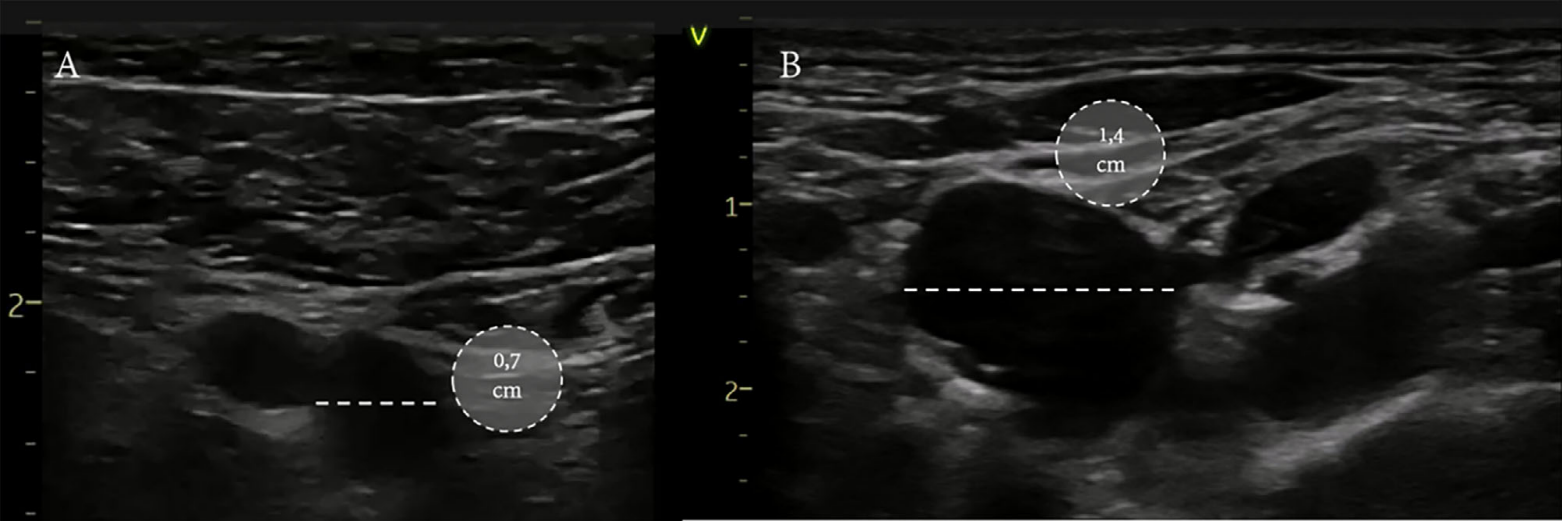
Ultrasound assessment of the right femoral vein (left: panel A) and right internal jugular vein (right: panel B) to evaluate vascular access for cardiac catheterization. [Colour figure can be viewed at wileyonlinelibrary.com]
